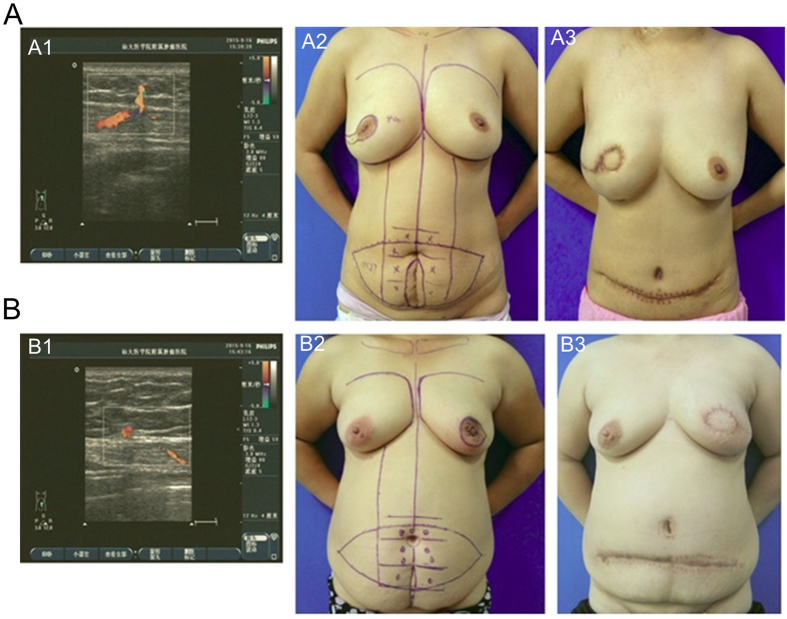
(A) A 37-year-old woman with invasive ductal carcinoma in the right outer quadrant close to the areola. A1: Location and peak systolic flow velocities of the perforators were assessed with pre-operative color-flow duplex ultrasound scanning. A2: The patient had a previous low midline abdominal scar and breast reconstruction with a single-pedicle transverse rectus abdominis myocutaneous (SP-TRAM) flap was planned. A3: The patient underwent mastectomy with immediate reconstruction using single-pedicle TRAM flap and was followed for 20 months. (B) A 50-year-old woman with invasive ductal carcinoma in the right central quadrant. B1: The perforator locations and peak systolic flow velocities were detected by pre-operative color-flow duplex scanning. B2: The patient had previous low midline abdominal scar and was scheduled for mastectomy with immediate SP-TRAM flap breast reconstruction. B3: The same patient at 6 months after surgery.
